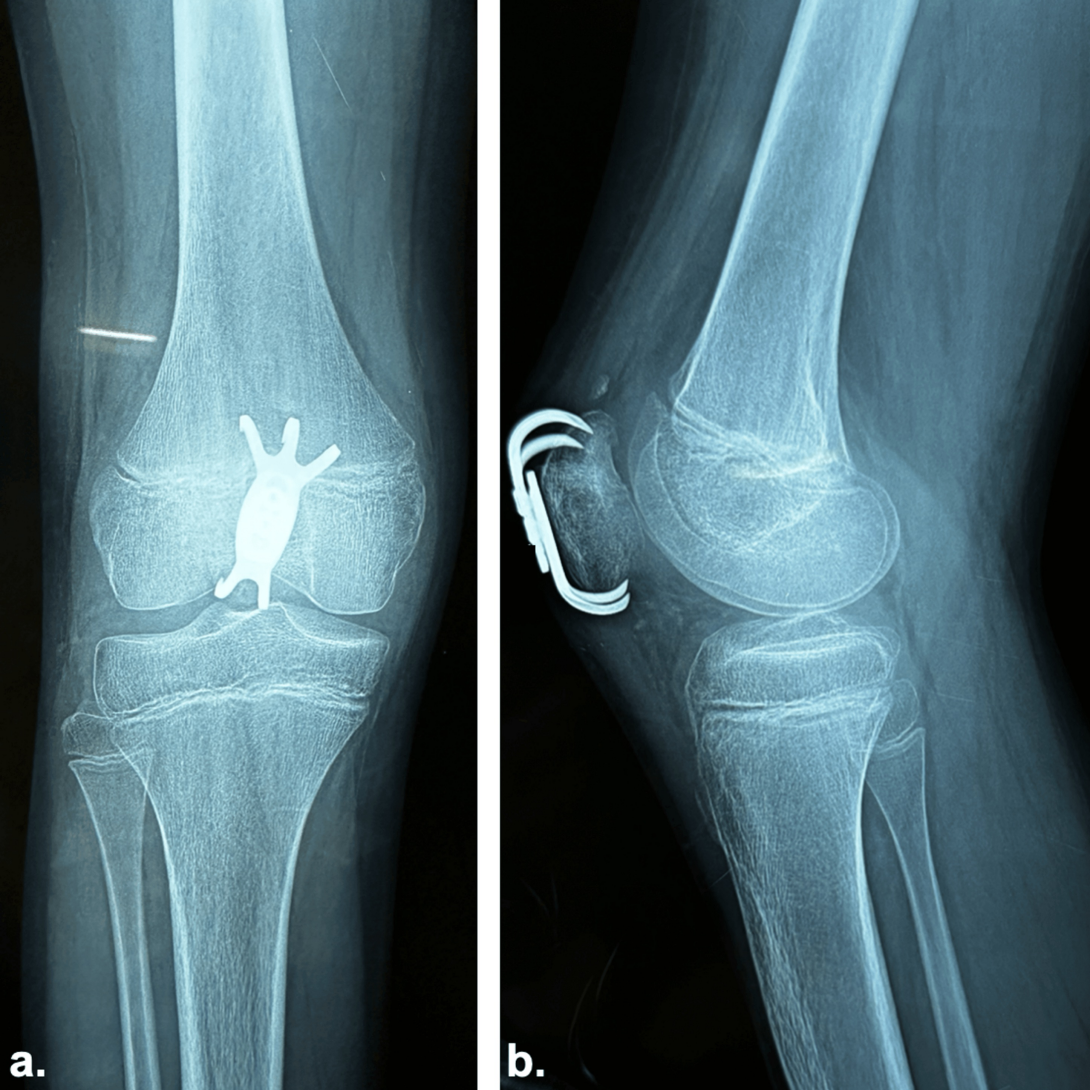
Postoperative X-ray at three months demonstrating fracture healing and restored patellar alignment a. Frontal view and b. Lateral view
